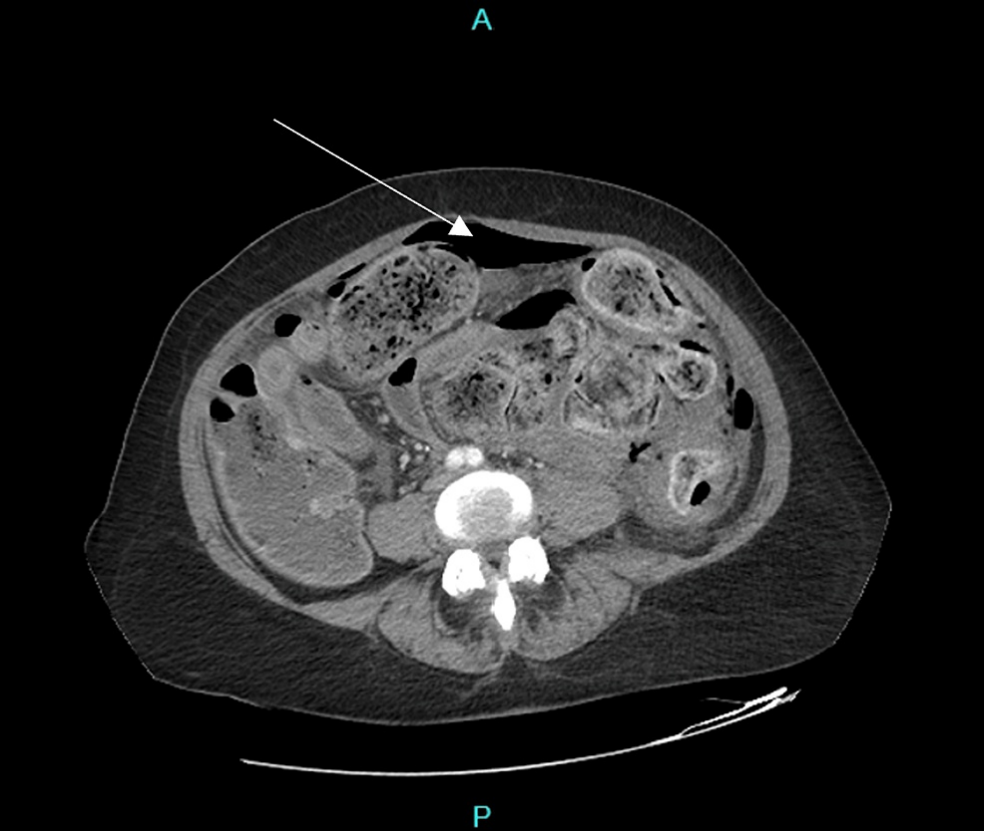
CT abdomen The image is showing peritoneal free air (arrow) and diffuse stool burden
